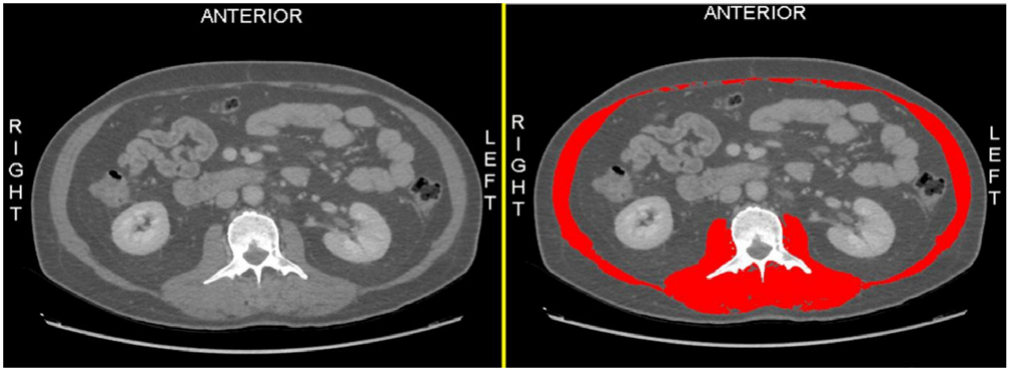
Computed tomography image for assessment of skeletal muscle area.
